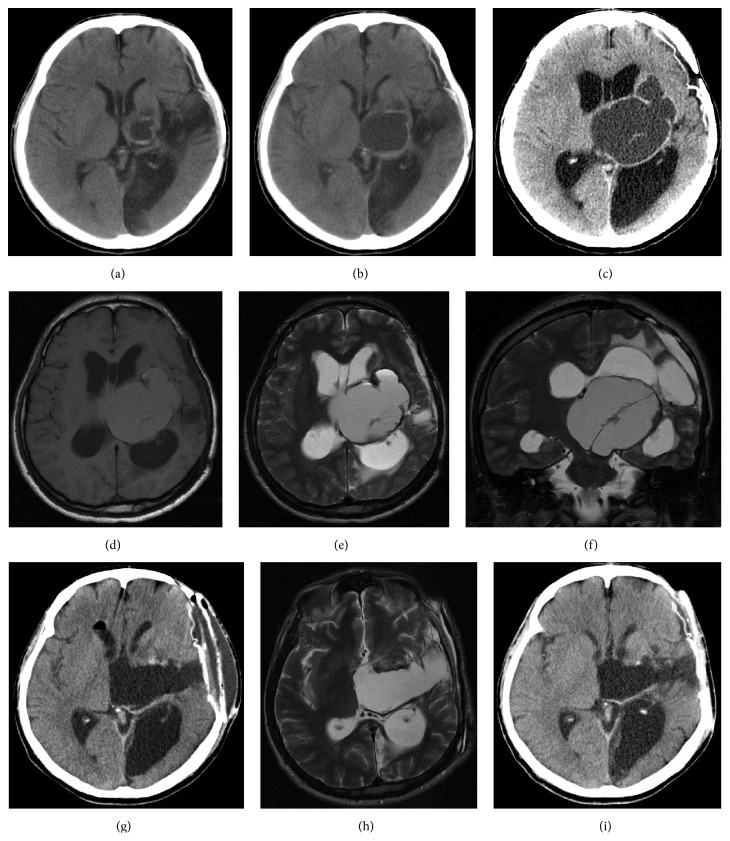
(a) Preoperative initial computed tomography (CT) 11 years after radiosurgery for an arteriovenous malformation in the left parietal lobe showing the cyst in the left thalamus. (b) Two years later, CT reveals that the size of the cyst is increased without hydrocephalus. (c) Four years later, CT reveals that the multilobular cyst is larger and accompanied by obstructive hydrocephalus. Preoperative magnetic resonance imaging (MRI) showing a cystic lesion in the left thalamus appearing isointense on T1-weighted imaging (d) and hyperintense on T2-weighted axial (e) and coronal (f) imaging as well as association with obstructive hydrocephalus. Note thickened cyst wall and septum in the middle of the cyst. Postoperative CT (g) and MRI (h) show shrinkage of the hematoma cavity and improvement of hydrocephalus. CT scan one year after surgery demonstrates neither recurrence of the hematoma nor progressive hydrocephalus (i).
